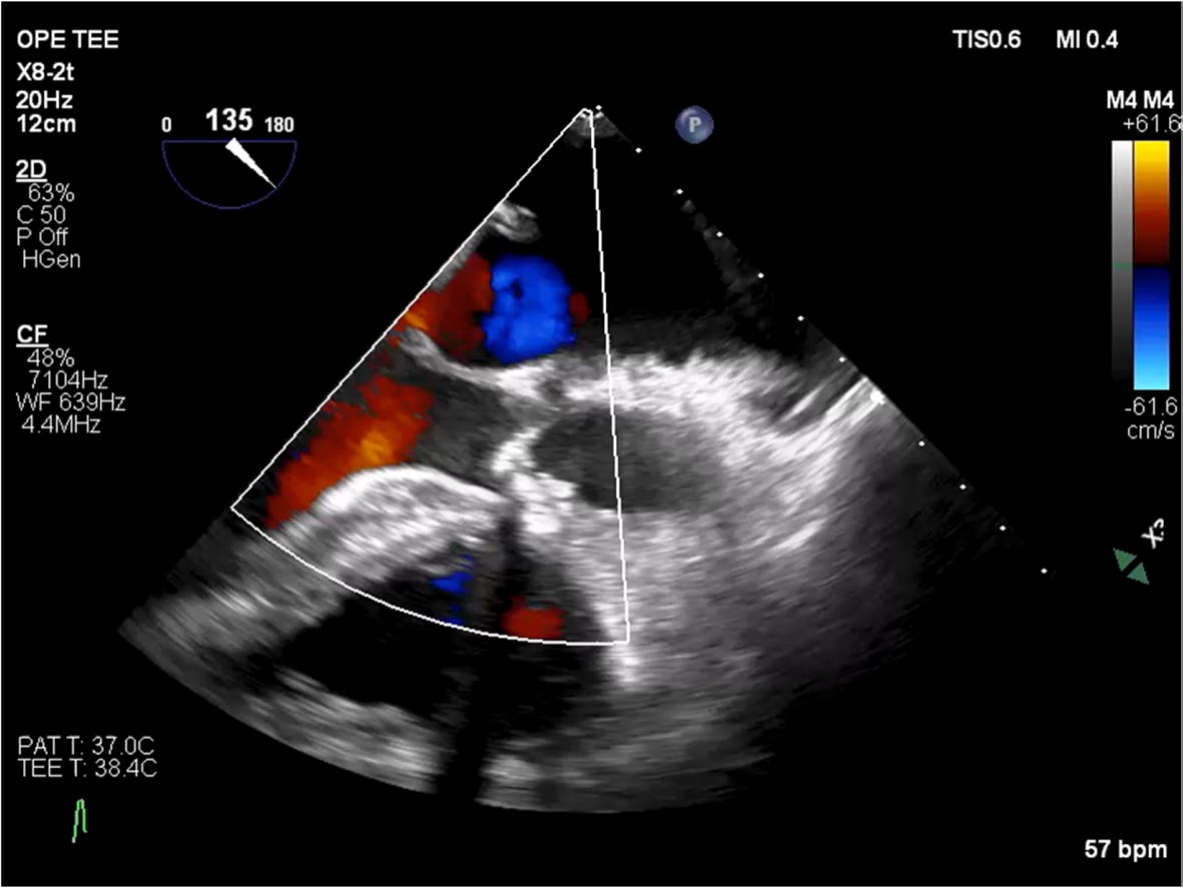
Intraoperative transesophageal echocardiography (TEE) (pre-deploy). Mid-esophageal long-axis view. Preprocedural examination shows trivial mitral regurgitation. The aortic valve was highly calcified, and transaortic valve color flow Doppler ultrasound shows a mosaic pattern of blood flow
